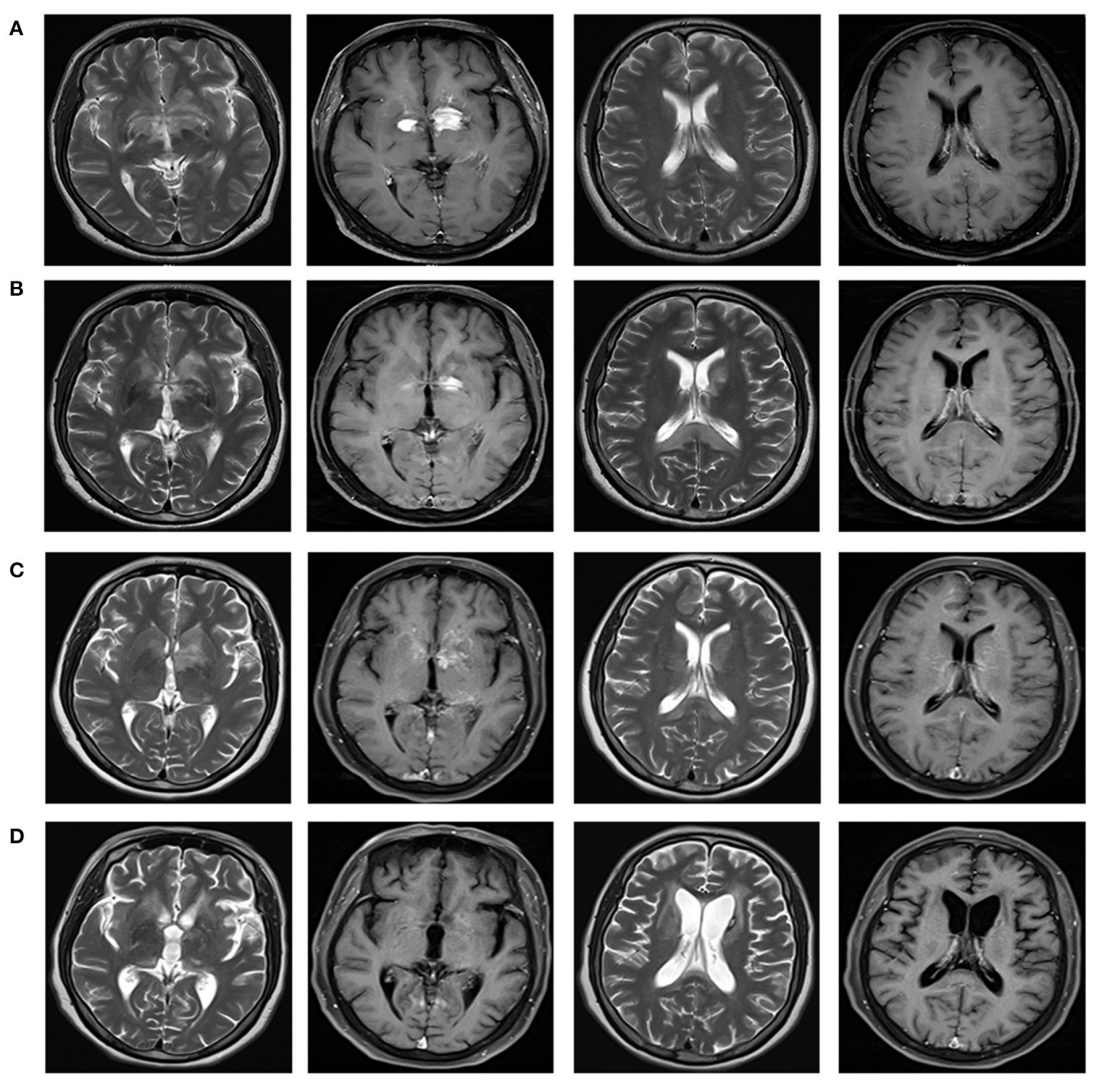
Brain magnetic resonance imaging reflecting the imaging changes of lesions in bilateral basal ganglia and around the third ventricle, and the changes of splenial corpus callosum lesions during the course of disease. Brain MRI with T2-weighted and T1 contrast-enhanced were shown in different columns. (A) In December 2020, multiple abnormal enhancement lesions in bilateral basal ganglia and around the third ventricle were demonstrated. (B) In January 2021, lesions in bilateral basal ganglia and around the third ventricle reduced significantly with less enhancement. A new T2 signal in the splenium of the corpus callosum without enhancement was shown. (C) In March 2021, lesions in bilateral basal ganglia and around the third ventricle were the same as before, with increased enhancement. Attenuated T2 signal abnormality in the splenium of the corpus callosum was observed. (D) In August 2021, lesions in the bilateral basal ganglia and around the third ventricle significantly reduced, with complete resolution of the enhanced lesions. Abnormal signal foci in the splenium of the corpus callosum disappeared.
